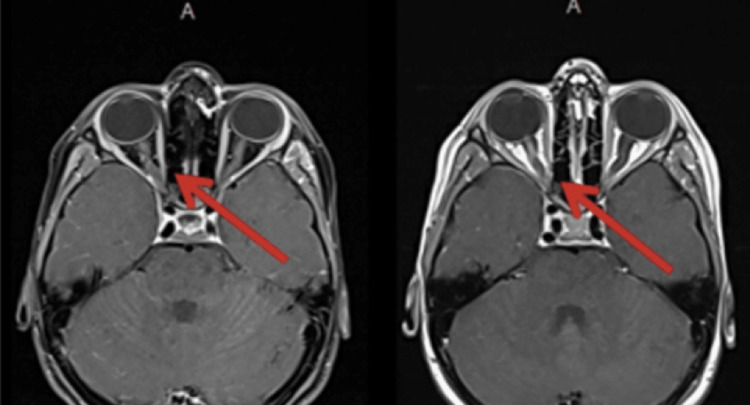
MRI of the brain showing the right optic nerve enhancement during a recurrent episode; MOG serology was positive at this time. MOG, myelin oligodendrocyte glycoprotein
